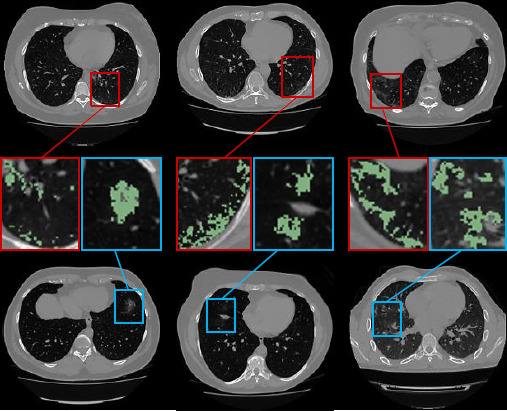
CT images of the lungs of a patient diagnosed with pneumonia. Available under the terms of the Creative Commons Attribution Non-Commercial License 4.0 [12].
